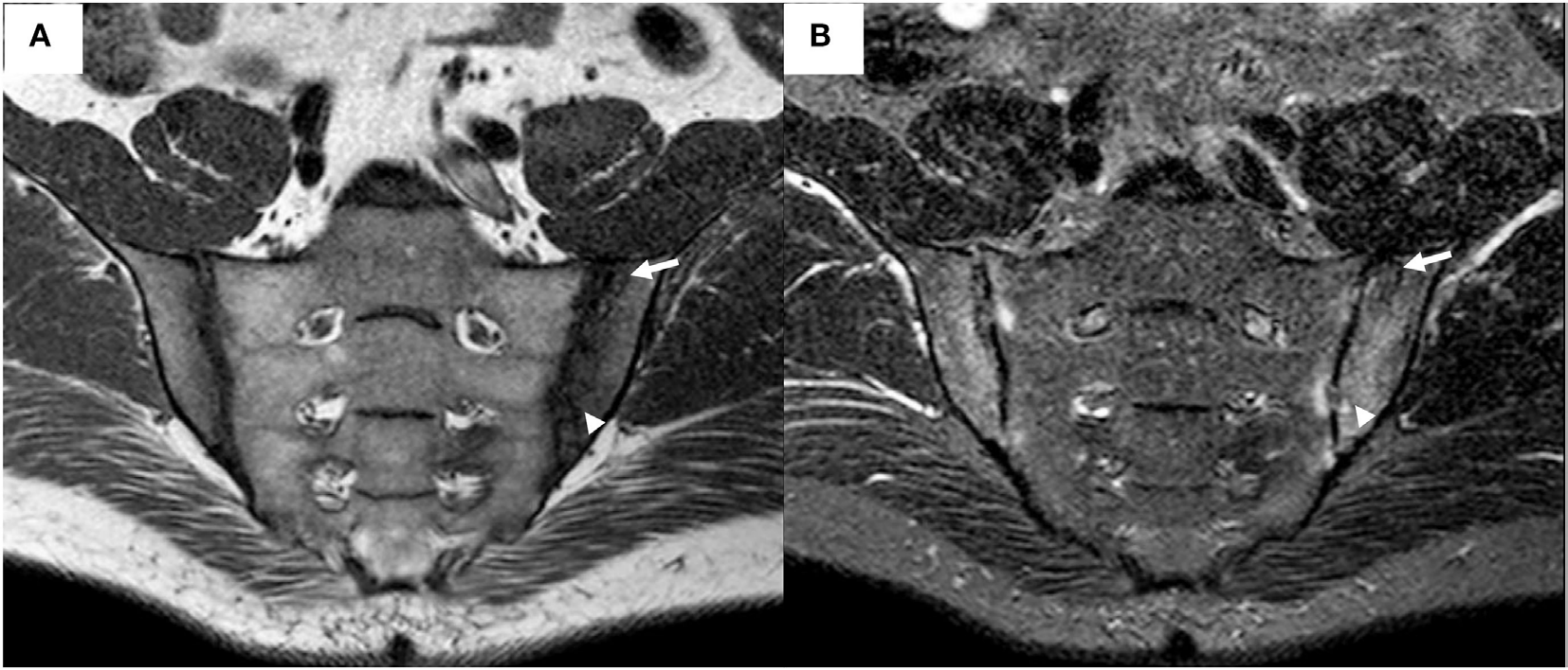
Magnetic resonance imaging (MRI) of the sacroiliac joints (same patient as depicted in Figure 1) demonstrates bilateral irregularity of the joint margins, and subchondral bone marrow edema appearing as a hypointense signal on T1-weighted (T1w) sequence (A) and as a hyperintense signal on short tau inversion recovery (STIR) sequence (B), fulfilling the Assessment of SpondyloArthritis Society criteria for positive MRI for sacroiliitis. An area of subchondral sclerosis with hypointense signal on both sequences (arrow), and an active erosion with hypointense signal on T1w sequence and hyperintense on STIR sequence (arrowhead), are visible in the left ilium.
